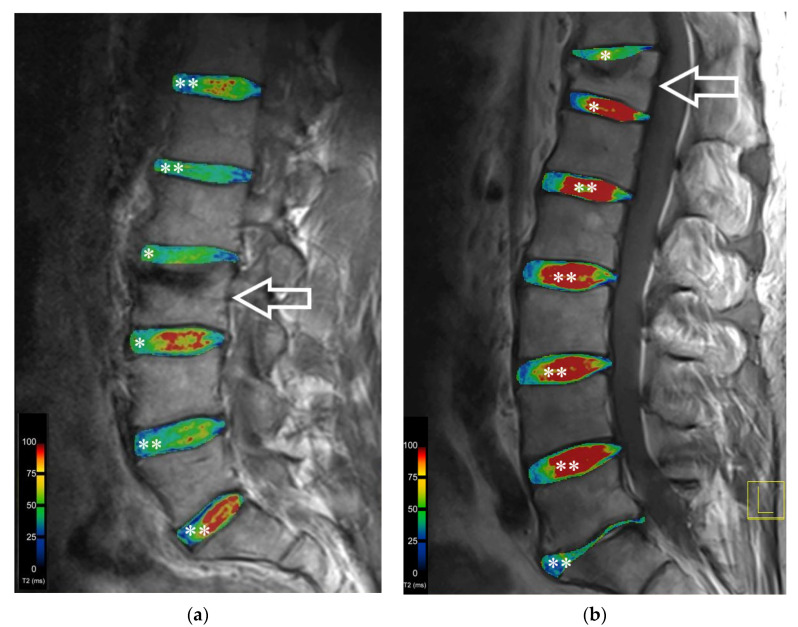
Sagittal TSE MR image and the corresponding colour-coded T2 map of the intervertebral disc. Sagittal TSE MR image and the corresponding colour-coded T2 map of the IVD of two different patients ((a) female, (b) male) show clearly visible differences between the upper intervertebral discs adjacent (*), especially upper IVD, and nonadjacent () to vertebral bodies after kyphoplasty. The arrow marks the vertebral body treated with kyphoplasty. The T2 values are represented by the colour-coded map within the IVDs, as shown in the colour bar.
